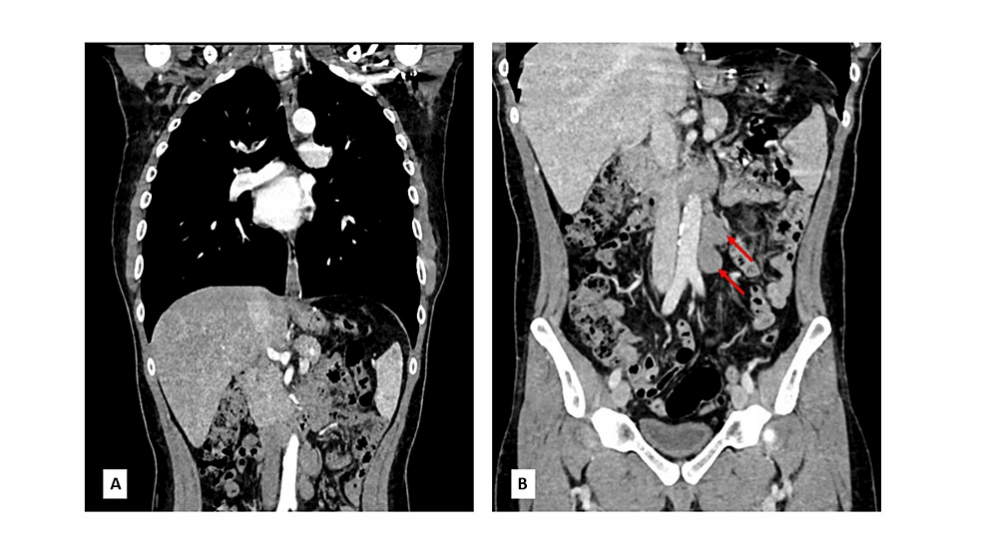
Staging chest, abdomen, and pelvis CT scan. A: Unremarkable CT of the chest with no metastatic lesions. B: CT of the abdomen showing two enlarged lymph nodes in the left para-aortic region (indicated by red arrows).
